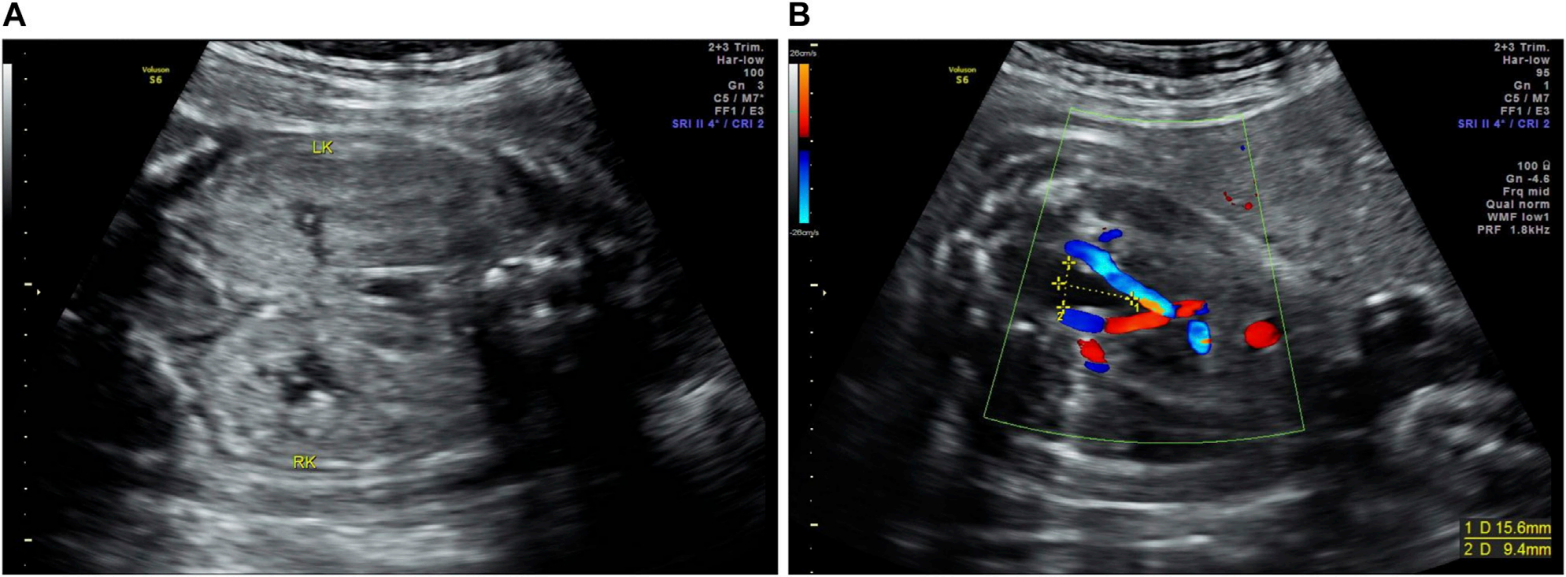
Prenatal ultrasound images of the proband (II-1). (A) The massively enlarged, echogenic kidneys of the proband (LK, left kidney; RK, right kidney). (B) The size of the bladder of the proband was 15.6 mm × 9.4 mm.
